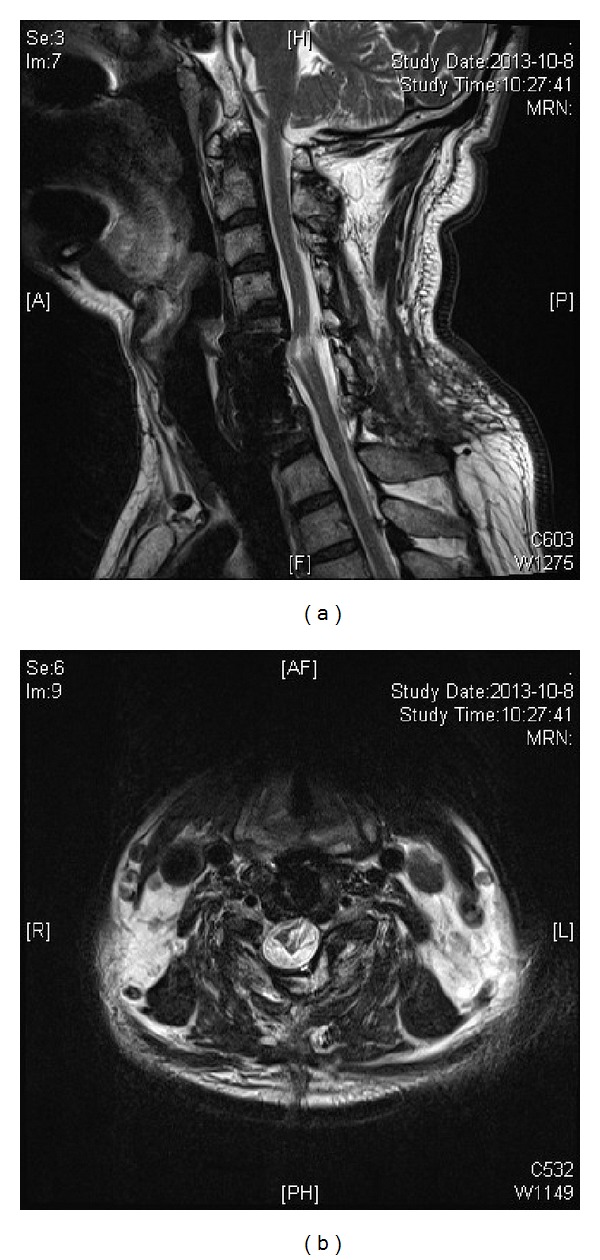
T2-weighted sagittal and axial magnetic resonance images made 3 months postoperatively. There is no stenosis, but persistent T2 high signal indicating myelomalacia is noted.
